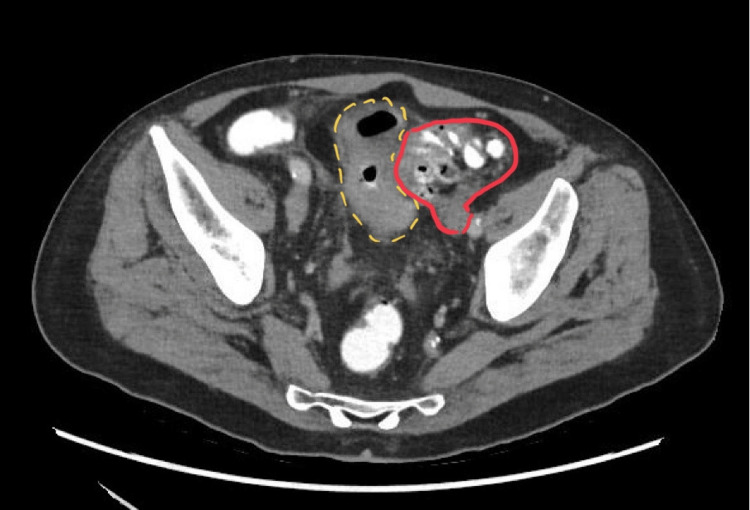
Axial slice computed tomography angiogram image demonstrating colovesical fistula. The bladder (with gas noted within it) is outlined by the yellow hatched line, and the sigmoid colon is outlined in red.
